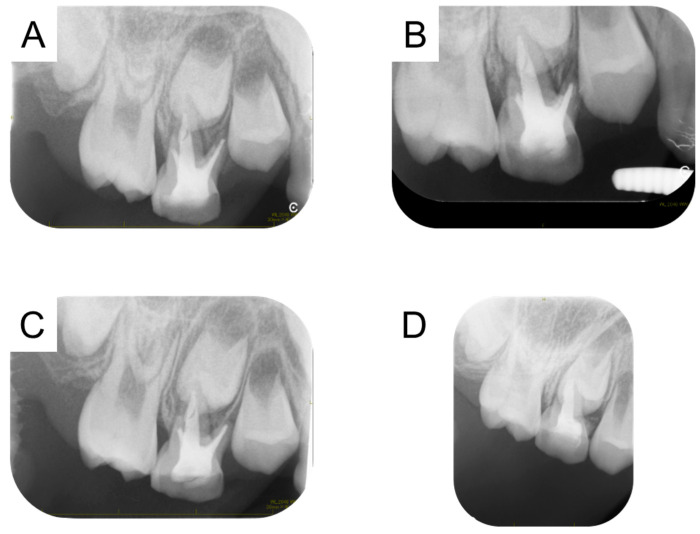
Radiographs of the maxillary right primary second molar: 7 years and 7 months (A), 7 years and 10 months (B), 8 years (C), and 8 years and 10 months (D).
